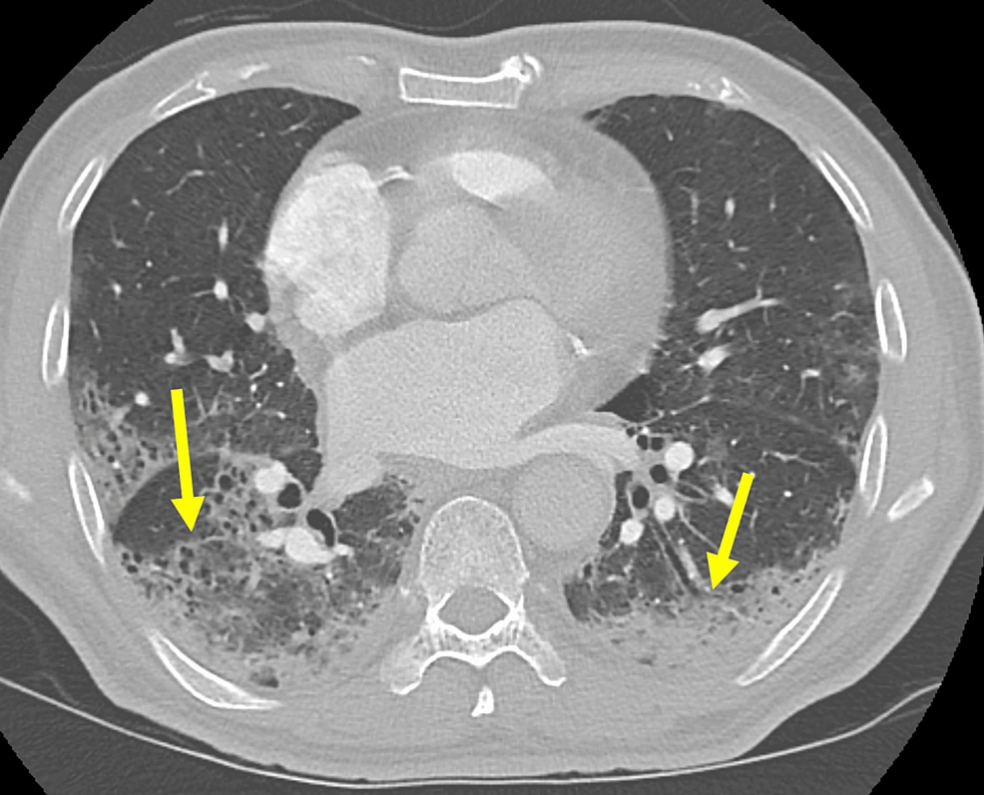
Bilateral peripheral ground-glass opacity and multifocal consolidations within the lungs on CT of the chest (arrows).
